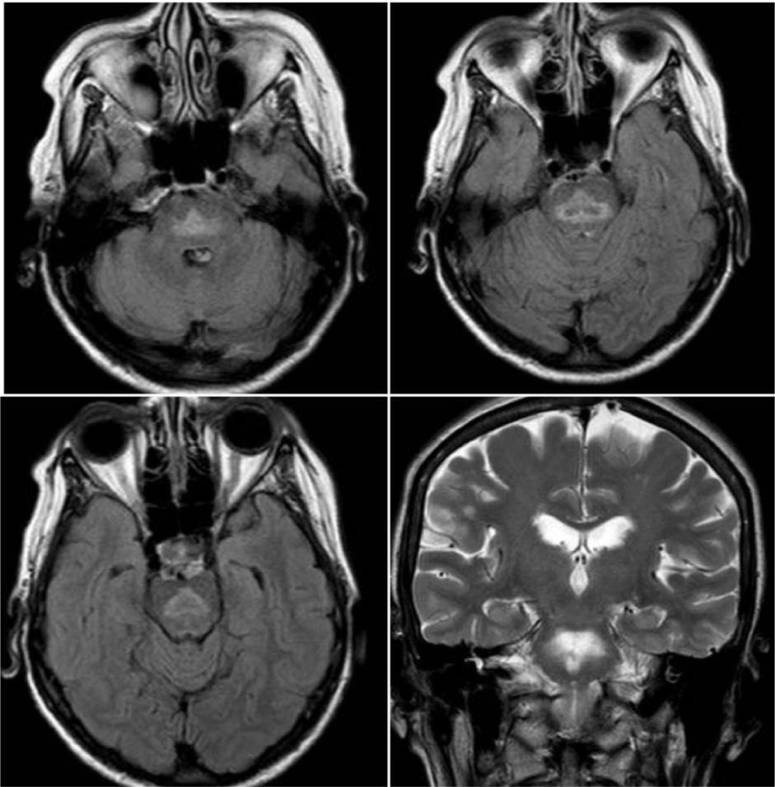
Axial FLAIR MR (a,b,c) and coronal T2 (d) weighted MR showing hyperintensity in the pons.
